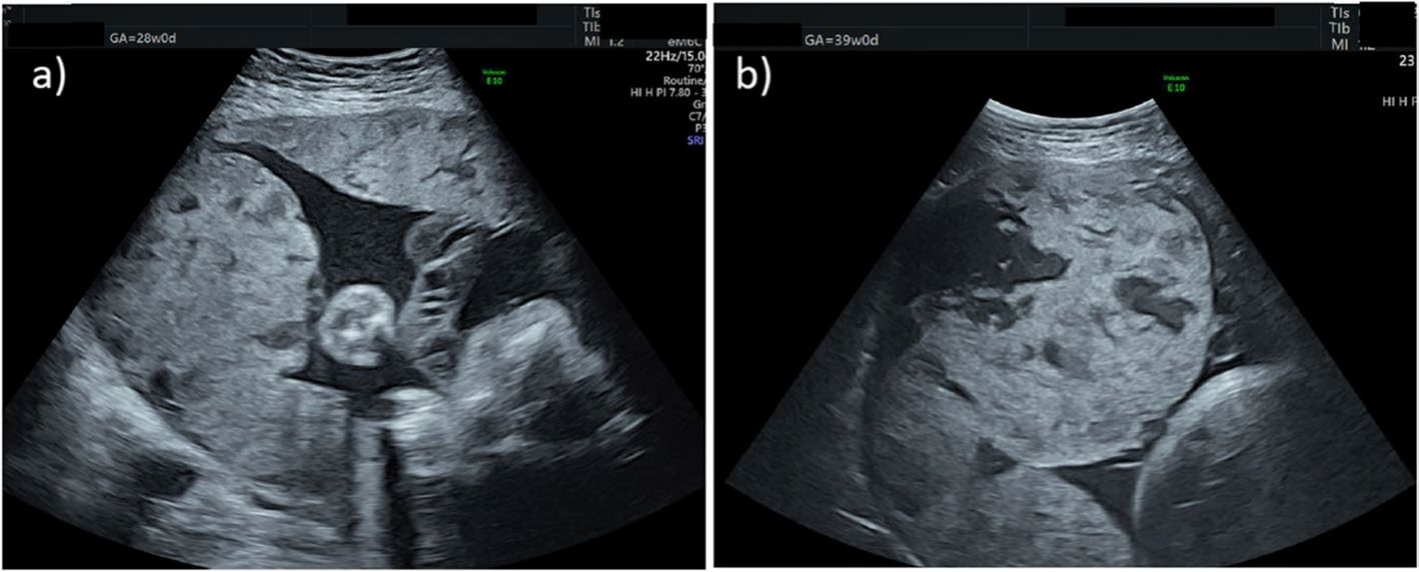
Ultrasonography image. a the placenta was at anterior to bottom of the uterus without clear borderline of the placenta and the muscle layer at the 28th gestational week. b Thickened placenta with large lacunae at the bottom of the uterus at 39th gestational week
